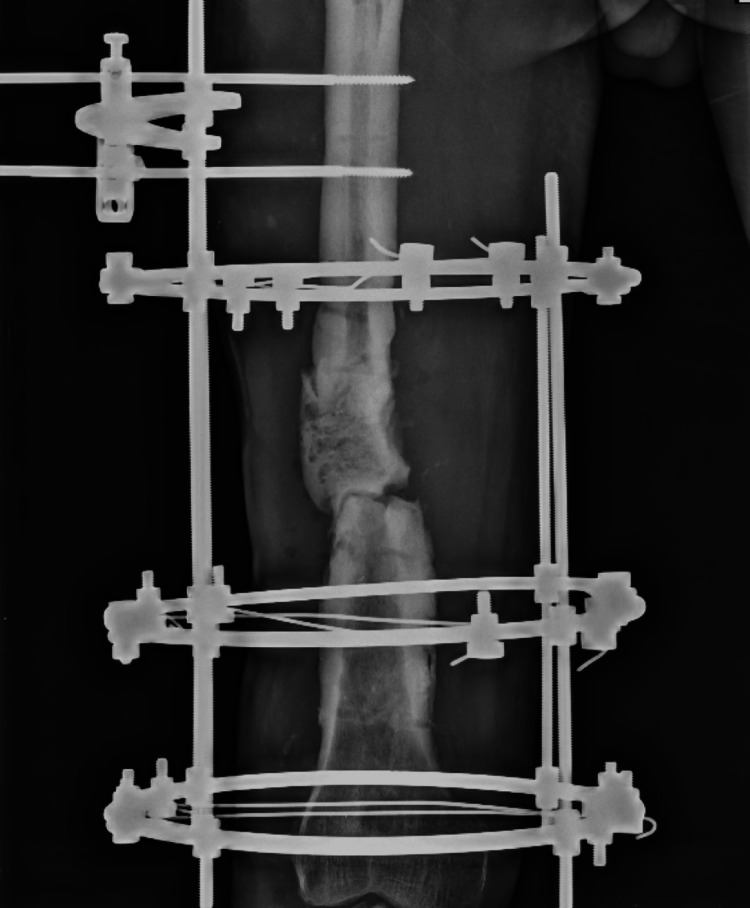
Ilizarov ring fixator X-ray
